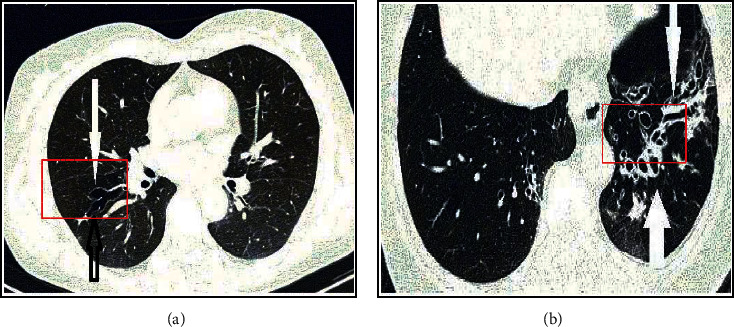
CT image of the patient's lungs.
